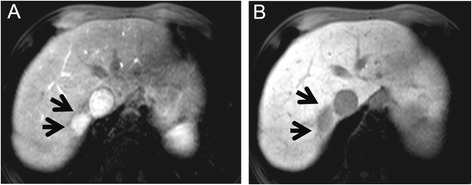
Contrast MRI showed a hyperintense nodule and hypointense nodule. A hyperintense nodule in the arterial phase (A) and a hypointense nodule in the hepato-biliary phase (B).
